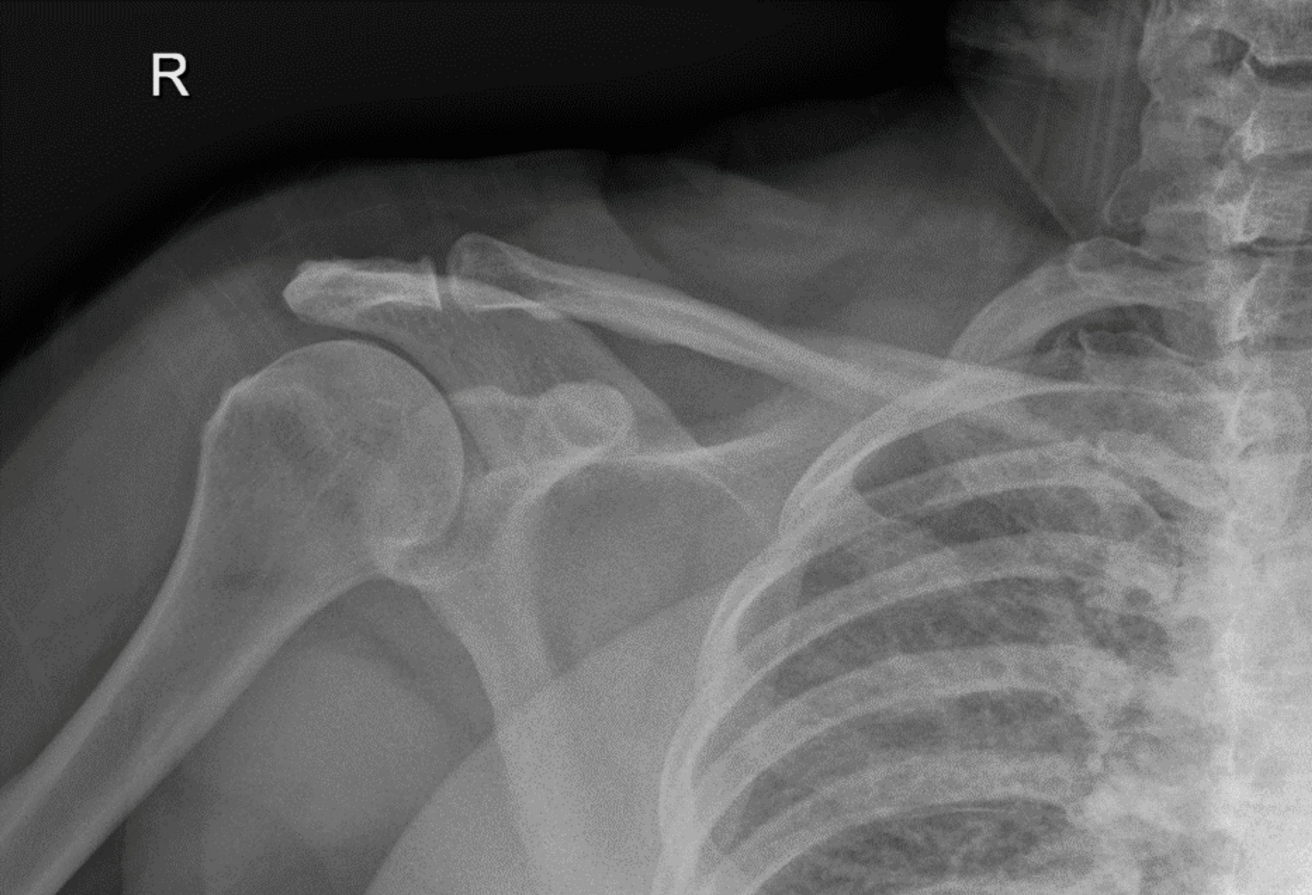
Case 1: Initial shoulder X-ray showing a right midshaft clavicle fracture, which appears to be non-displaced
